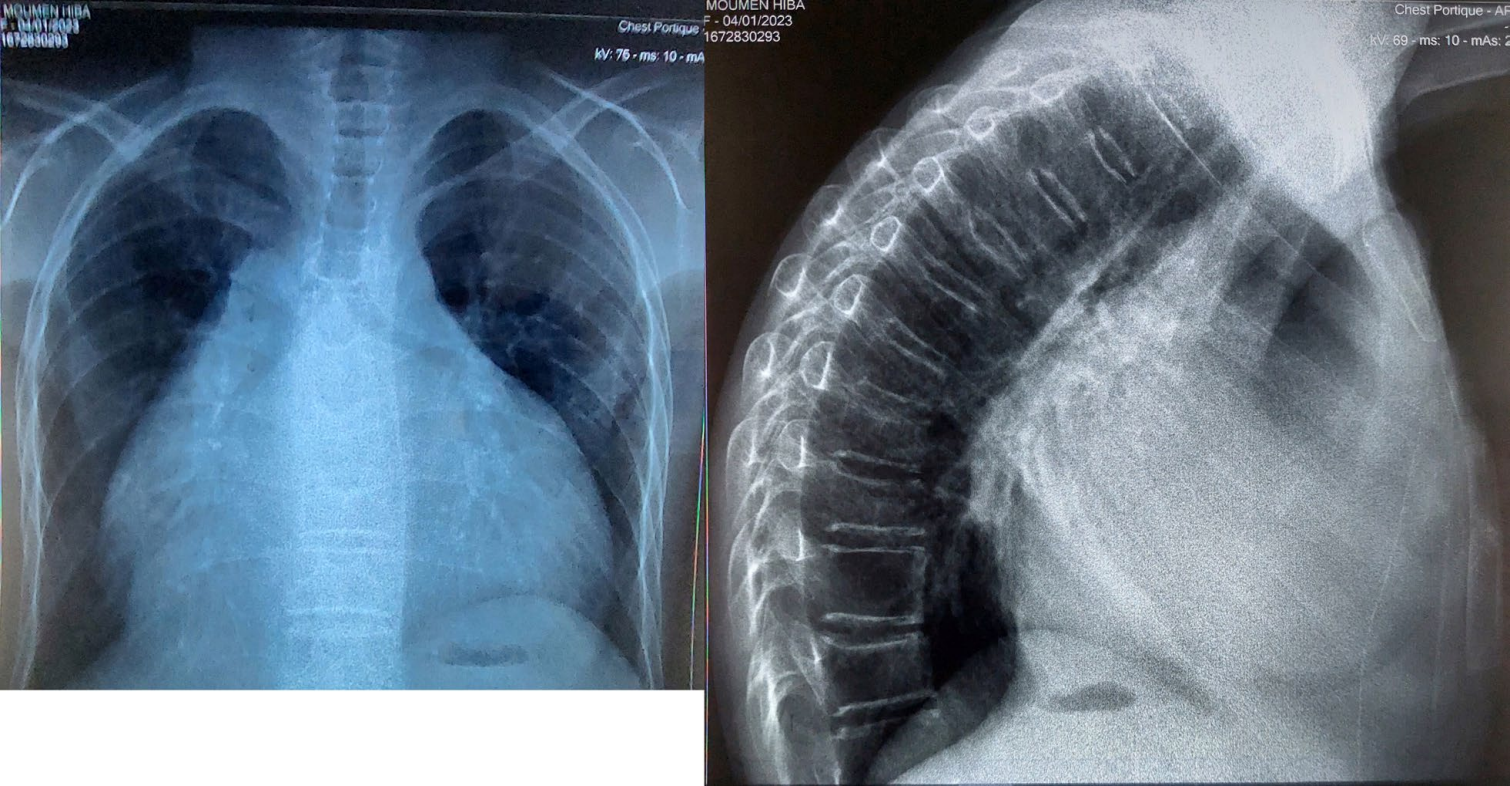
Chest X-ray front and profile
